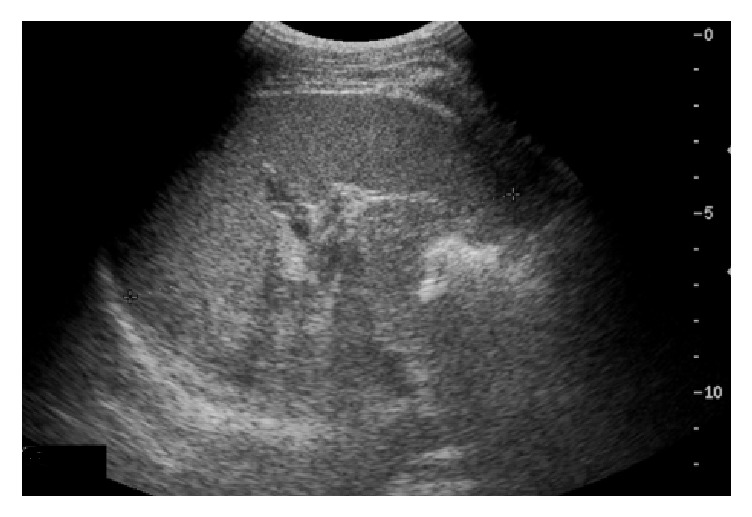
After six months of ATT, ultrasound showed a normal spleen.
